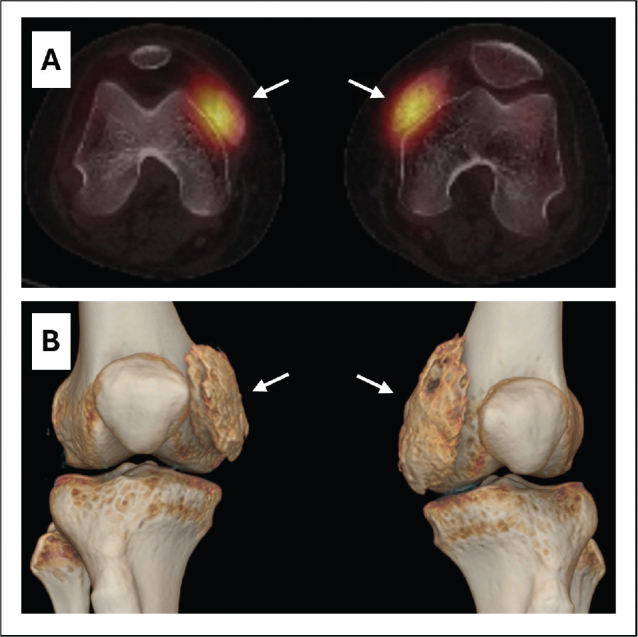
(A) Transversal SPECT-CT of the knees with fused images showing active bilateral heterotopic ossifications 6 weeks after first symptoms. (B) 3D reconstructed image showing the localization of heterotopic ossifications with regard to the knee joint.
